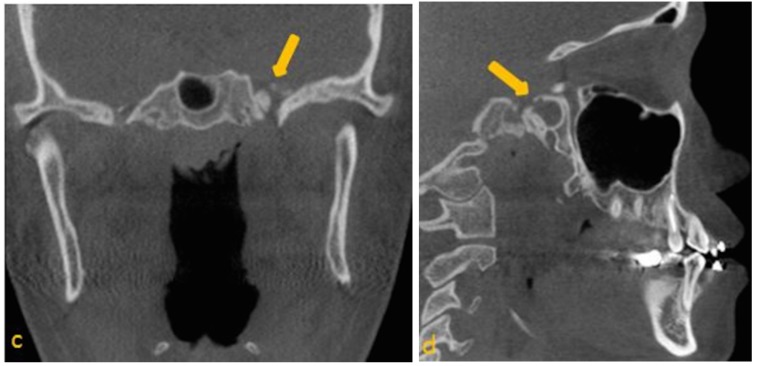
(a) Para-axial; (b,c) Paracoronal and (d) Parasagittal CBCT images through sphenoid Show low-attenuation area in the greater wing of sphenoid on the left side. (a) Para-axial image, depicts suspected disruption of the superior lateral wall of the carotid canal (arrow head); (b) Paracoronal view, note at the close proximity of entity to the vidian canal; (c) Paracoronal image, note at the irregular border of the left foramen ovale and presence of calcification; (d) Parasagittal view, shows extension of entity to the left pterygoid process.
